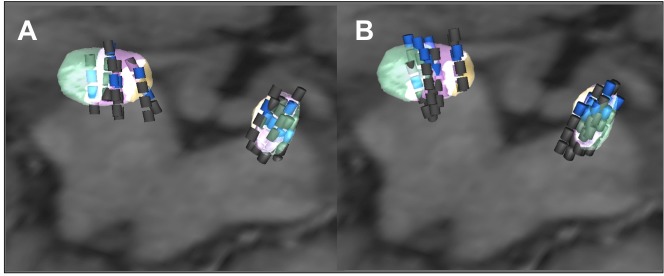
Electrode locations on postoperative MRI in the 17 PD patients operated for STN-DBS. Superior view of the STN after fusion with the three-dimensional MRI acquisition, an axial plane of which is shown. Electrode localisation is presented along the long axis of each electrode with transparent rendering of the three STN territories (limbic, yellow; associative, purple; motor, green). The active contacts are blue and the non active contacts are grey. Using this strategy, the mean mediolateral, anteroposterior and dorsoventral coordinates of active contacts in the 17 PD patients were 11.4±1.5 mm, 9.3±1.4 mm and 3.0±1.7 mm, in the anterior commissure-posterior commissure (AC-PC) space respectively. Panel A shows the electrode locations for the 7 patients who received the perioperative psychoeducation programme. Panel B shows the location of the electrodes in the 10 patients without perioperative psychoeducation programme.
